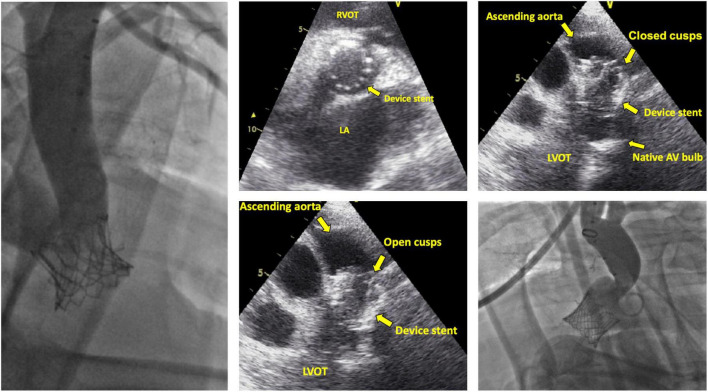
Fluoroscopy and echocardiography images of the implanted prosthesis.
